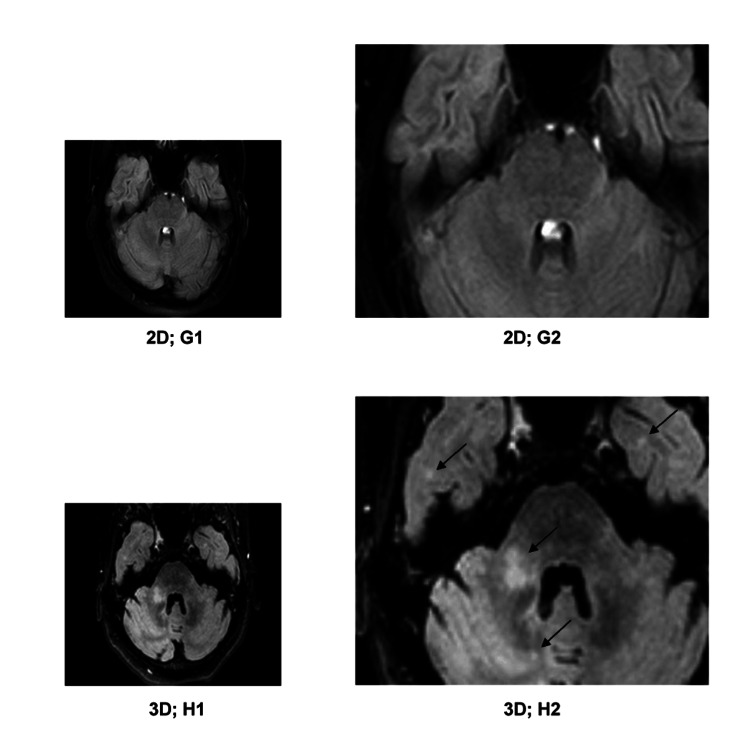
G1) 2D-FLAIR image; G2) detailed enlargement of G1; H1) 3D-FLAIR image, approximately corresponding to G1; H2) detailed enlargement of H1. On the 3D-FLAIR images, white matter lesions in the right middle cerebellar peduncle and bilateral temporal lobes (marked with arrows in H2) are visible, which remained undetected on the 2D-FLAIR images.
